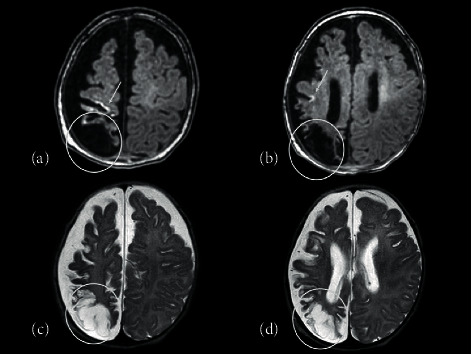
Head MRI. (a, b) T1-weighted MRI; (c, d) T2-weighted MRI. Parasagittal cerebral injury and ulegyria in the right parietal lobe and occipital lobe were observed. Arrows indicate parasagittal injury and circles indicate ulegyria.
